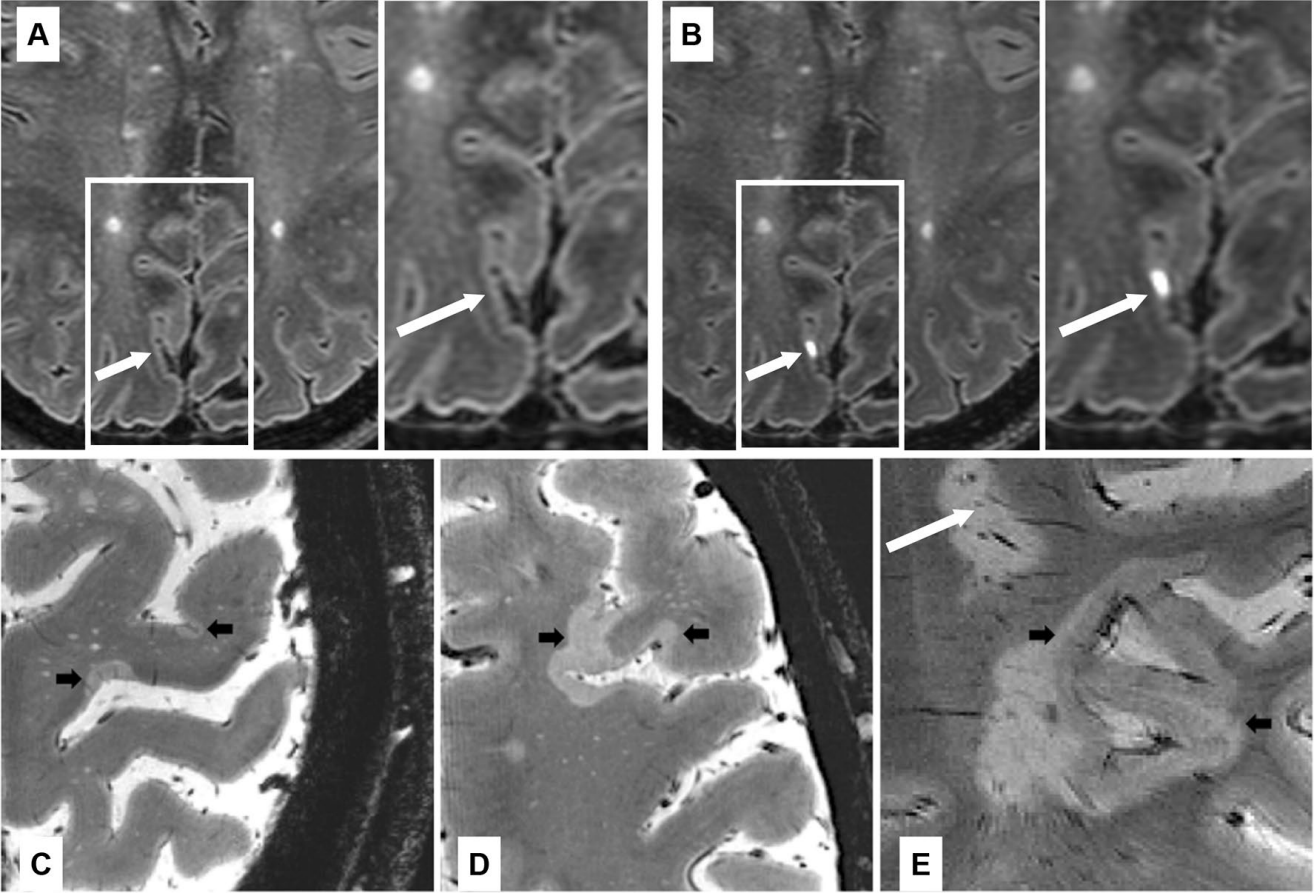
Leptomeningeal enhancement, cortical lesions and subpial demyelination. A, B Example of 7T FLAIR MRI of the brain in a 49-years-old woman with relapsing–remitting multiple sclerosis before (A) and after (B) the administration of gadolinium-based intravenous contrast agent. A focus of post-contrast pial/subarachnoid enhancement highlighted by white arrow. Examples of focal (C) or more extensive (D, E) subpial multiple sclerosis lesions (black arrows) with expansion within white matter/confluence with a juxtacortical lesion (E) in patients with multiple sclerosis as seen on ultrahigh resolution T2* gradient echo images at 7 Tesla. A white matter lesion is also visible in (E) (white arrow). Abbreviations: *FLAIR* fluid-attenuated inversion recovery, *MRI* magnetic resonance imaging
